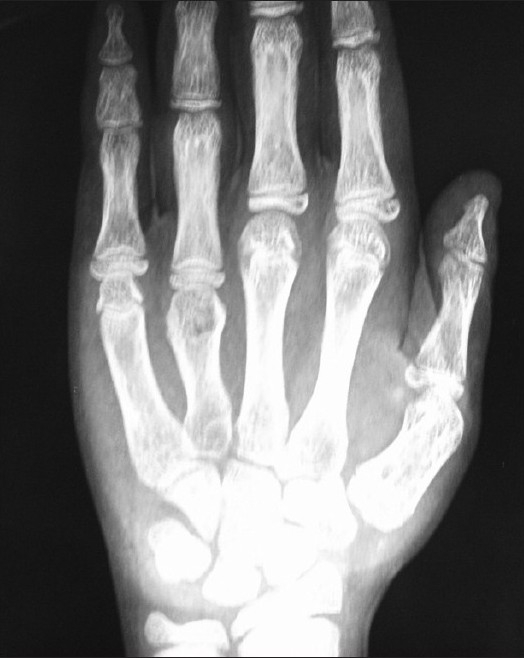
Photograph shows X-ray of hand AP view with short fourth metacarpal bone.
